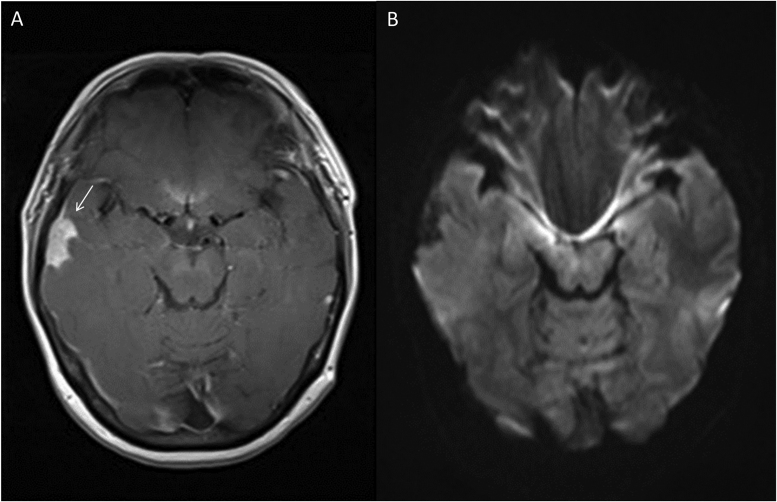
(A) Axial T1 magnetic resonance imaging scan of the brain post gadolinium demonstrating a solid right temporal lesion with enhancement (indicated with a white arrow). (B) Diffusion weighted magnetic resonance imaging scan of the axial brain with no evidence of restricted diffusion.
